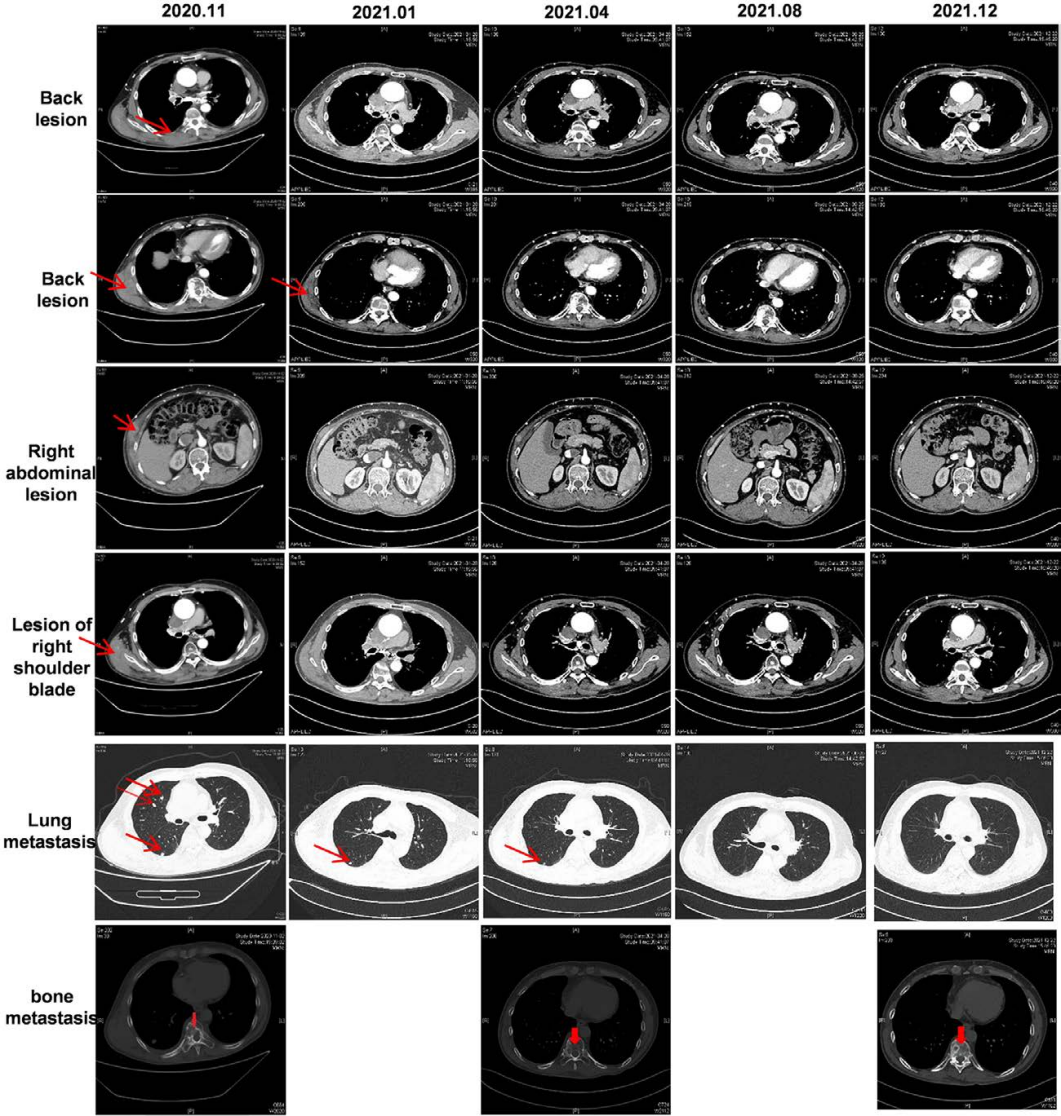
CT images during treatment, including back, lung, and bone metastasis, the red arrow indicates the location of the lesions. CT = computed tomography.
